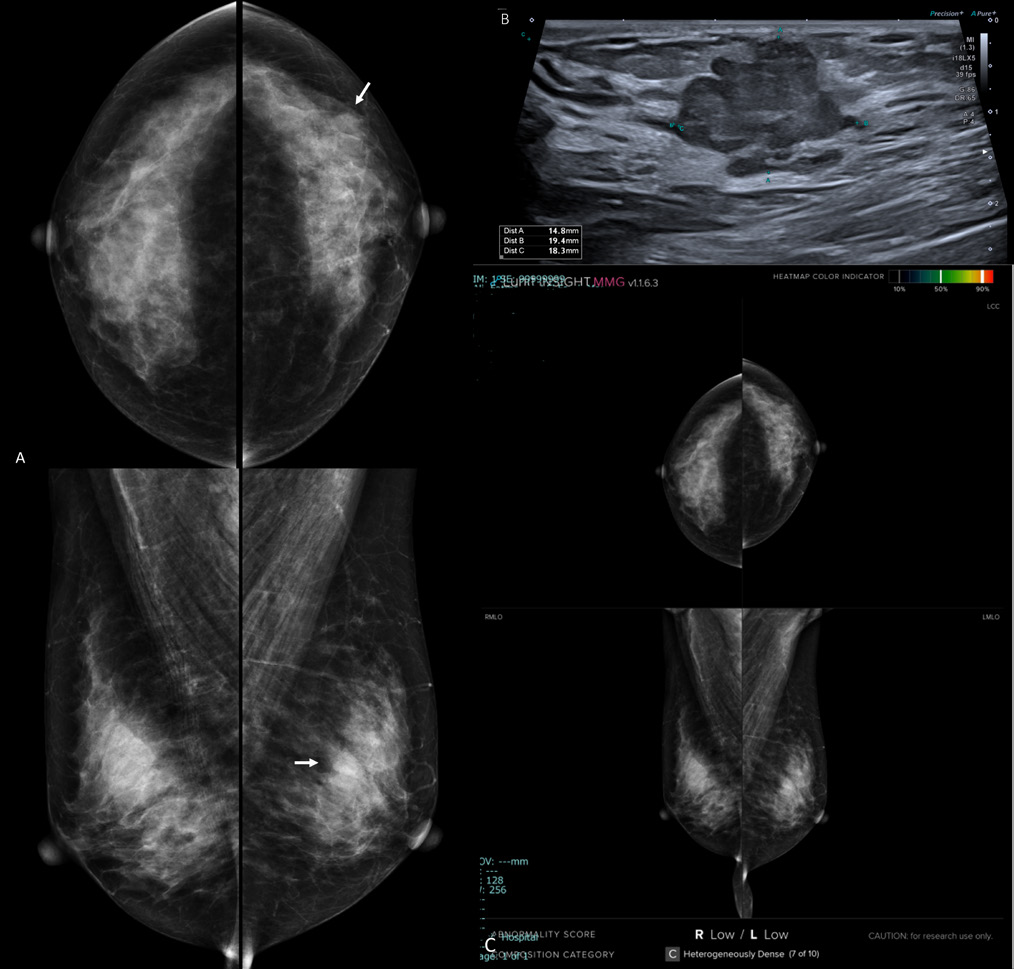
Female patient 50 years old with heterogeneously dense breasts (ACR c) and diagnosed by left breast mucinous carcinoma grade I. A: bilateral digital mammogram (cranio-caudal and medio-lateral oblique views). There is a left breast upper outer suspected mass predominantly obscured by glandular tissue (arrow). B: Ultrasound image displayed irregular solid mass of fat near echopattern and in plane with the breast tissue. C: Artificial intelligence scanned 4 view mammograms displayed no visual color overlying and “Low” (i.e., < 10%) abnormality scoring suspicion of malignancy
